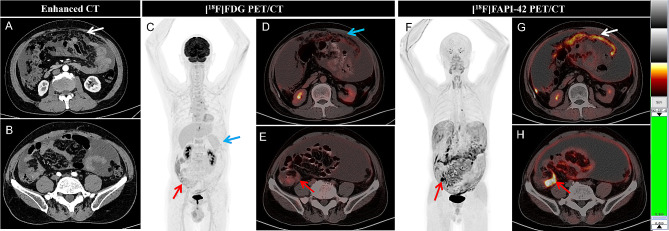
A 72-year-old man presented with abdominal distension for more than one month. The axial CE-CT suggested peritoneal tuberculosis (A, white arrow) and a large amount of ascites (A, B). ^18^F-FDG PET MIP showed no abnormal activity in the whole body (C), but the axial fused images showed mild ^18^F-FDG uptake in the omentum majus (SUVmax, 2.2, D, white arrow) and appendix (SUVmax, 4.7, E, red arrow). However, the MIP of ^18^F-FAPI-42 PET showed intense FAPI uptake in the abdominal (F, red arrow). The axial fused images showed intense FAPI uptake in the omentum majus (SUVmax, 11.8, G, white arrow) and appendix (SUVmax, 19.7, H, red arrow). Appendiceal neoplasms with peritoneal metastasis was diagnosed. Then the patient underwent abdominal exploration and was confirmed to be a poorly differentiated adenocarcinoma contained signet ring cell carcinoma by biopsy of omental node. ^18^F-FAPI-42 PET/ CT was more sensitive to detect the primary tumor and peritoneal metastasis than ^18^F-FDG PET/ CT and enhanced CT and changed the treatment plan compared to CE-CT
